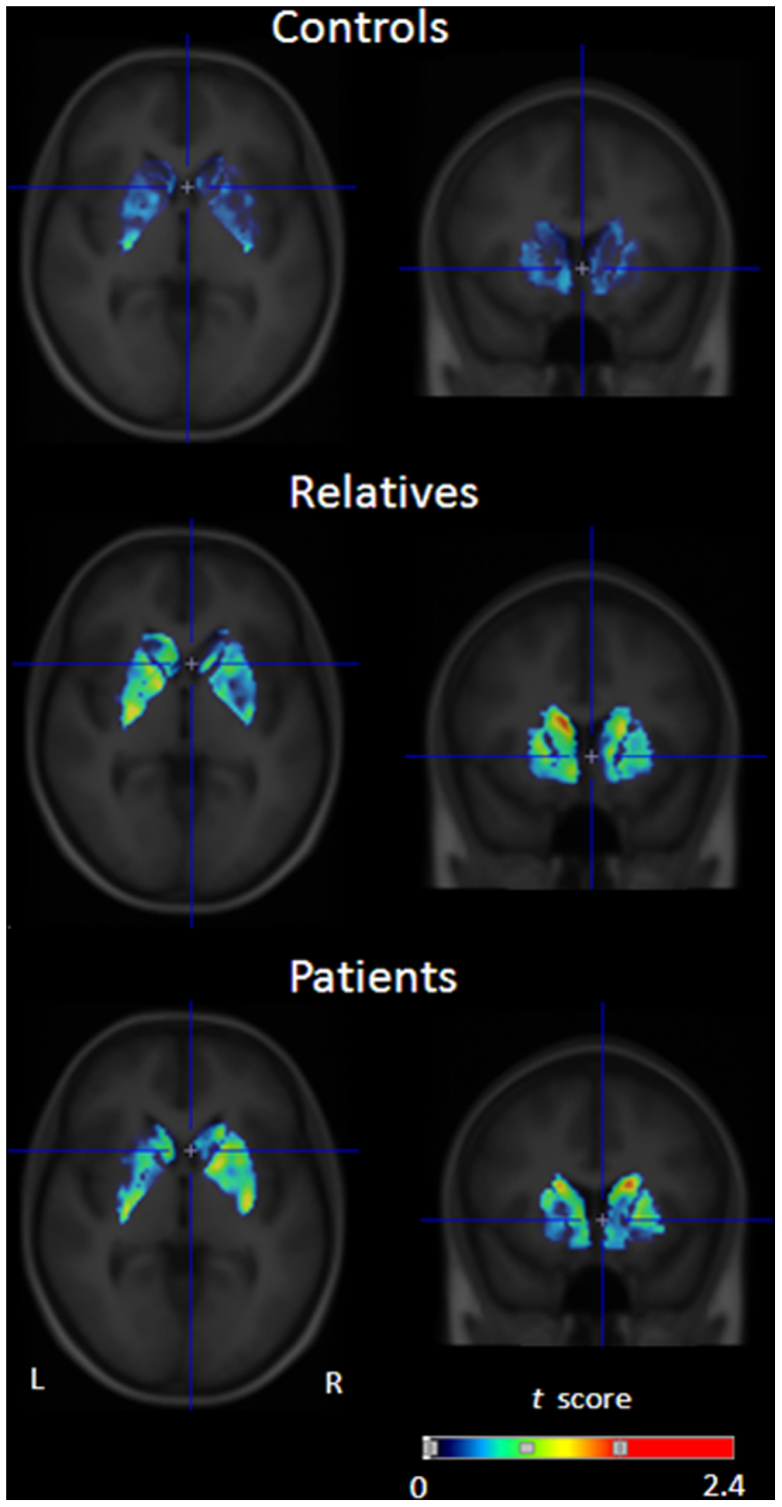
Mean statistical parametric *t* map of γ in sagittal (left) and coronal (right) sections overlaid on a MRI template, showing Δ^9^-THC-induced ^18^F-fallypride displacement at the level of the striatum (x = 0, y = 11, z = −4) for controls (top row, n = 9), relatives (middle row, n = 8) and patients (bottom row, n = 7, one subject was excluded from this analysis due to anomalous (non)physiological values). The image is thresholded for visualization purposes (maximum t obtained for controls, relatives and patients, respectively: 1.15, 2.6, and 2.7).
